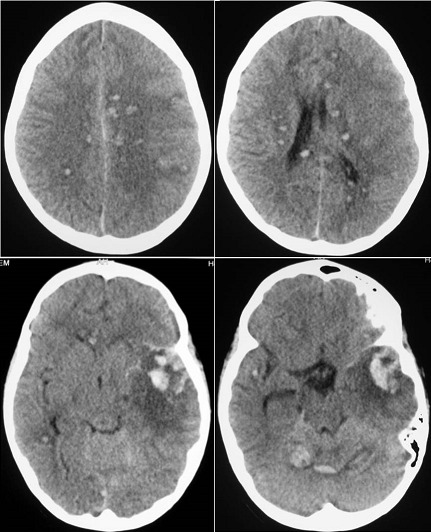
Cranial computed tomography scan showing disseminated intracerebral hemorrhage
